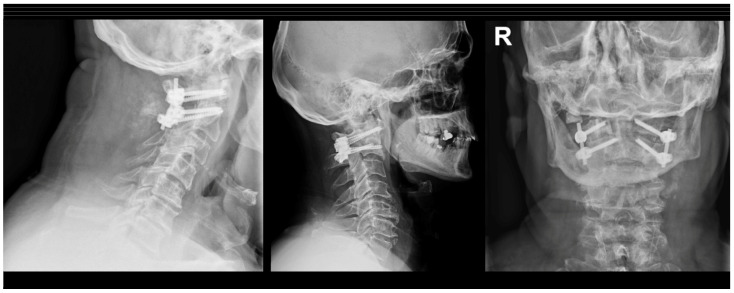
Postoperative standing X-rays after C1–C2 fusion.
